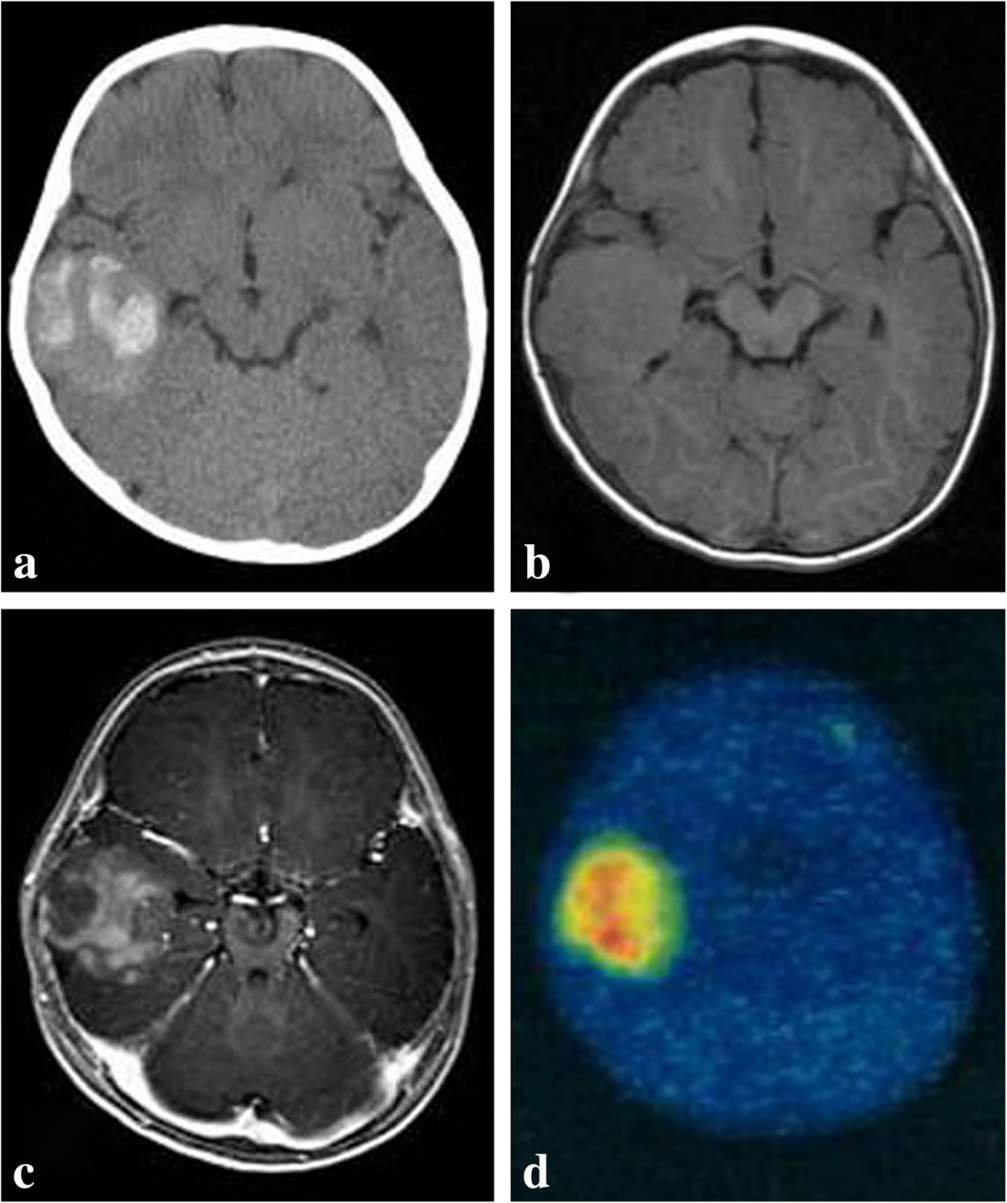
Radiological imagings at admission. (a) Computed tomography scan showing a calcified lesion in the right temporal lobe. Magnetic resonance imaging scans (b): T1 plain-weighted image, (c): Gadolinium-diethylenetriamine pentaacetic acid [Gd-DTPA]-enhanced images) showing a mass in the left temporal lobe. The mass was heterogeneously enhanced with Gd-DTPA. (d) Positron emission tomography showing high uptake of methionine in the lesion.
